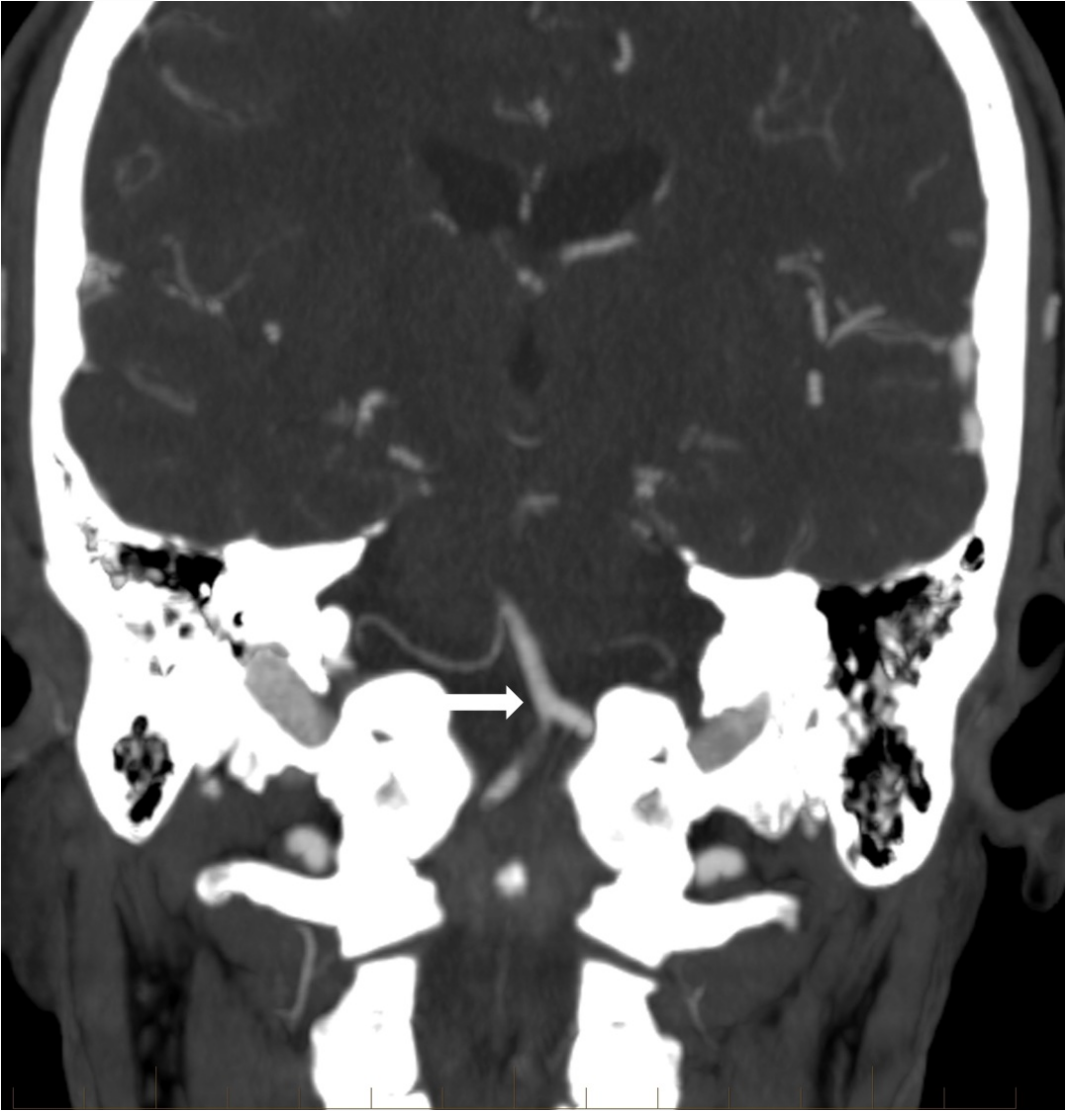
Repeat computed tomography (CT) angiogram after oral antiplatelet therapy and intravenous heparin reveals resolution of the partially occlusive thrombus in the proximal basilar artery (arrow).
